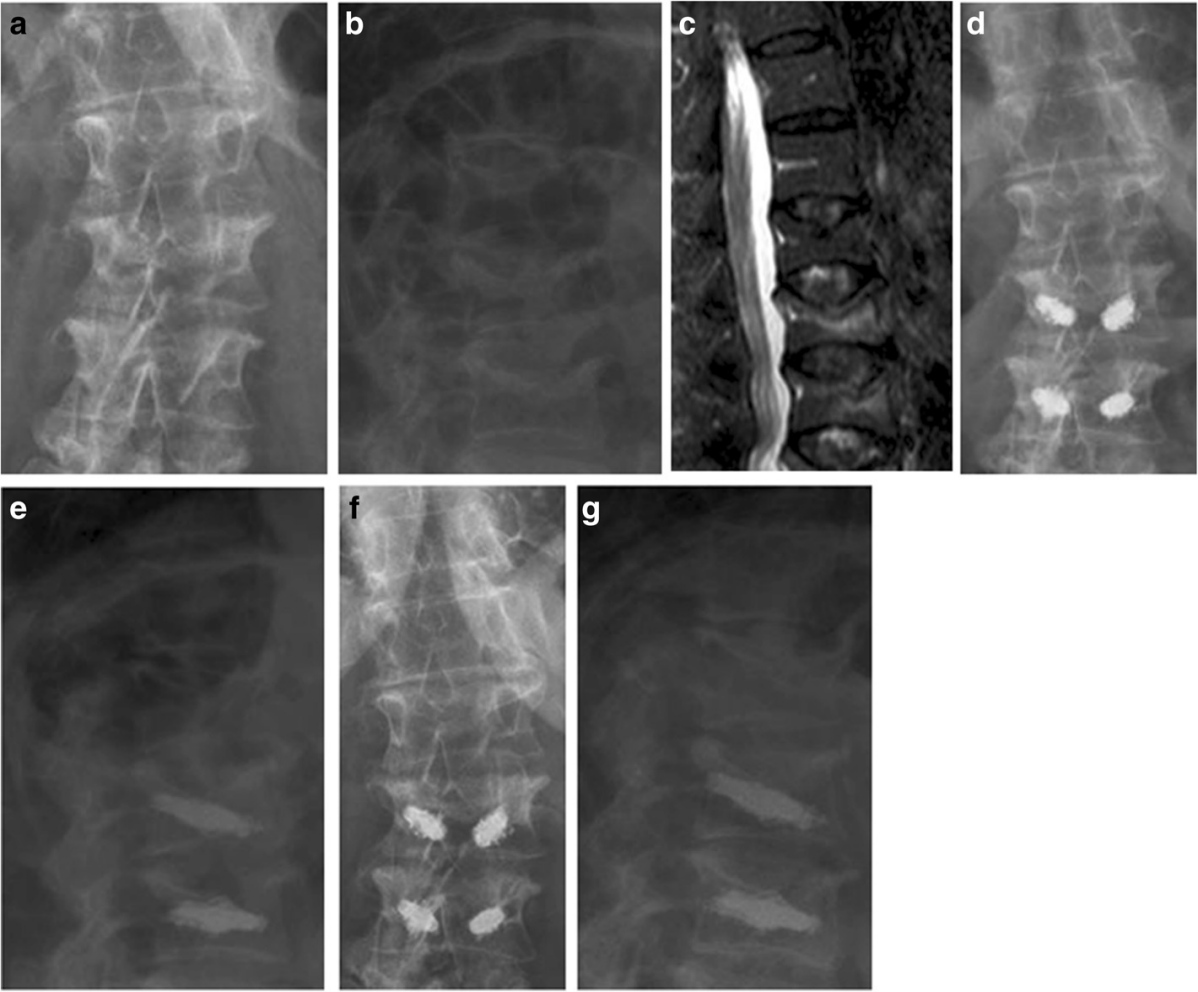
a–g Clinical case with X-ray imaging and MRI. Legend: Preoperative (a–c), postoperative (d, e) and X-ray imaging as well as MRI after 12 months (f, g) of an osteoporotic incomplete L2 burst fracture and L3 compression fracture without involvement of the posterior wall and stabilization using two cement-augmented titanium mesh cages (preoperative VAS 10.0, ODI 70.0 %; 12 months postoperative VAS 2.0, ODI 34.0 %)
